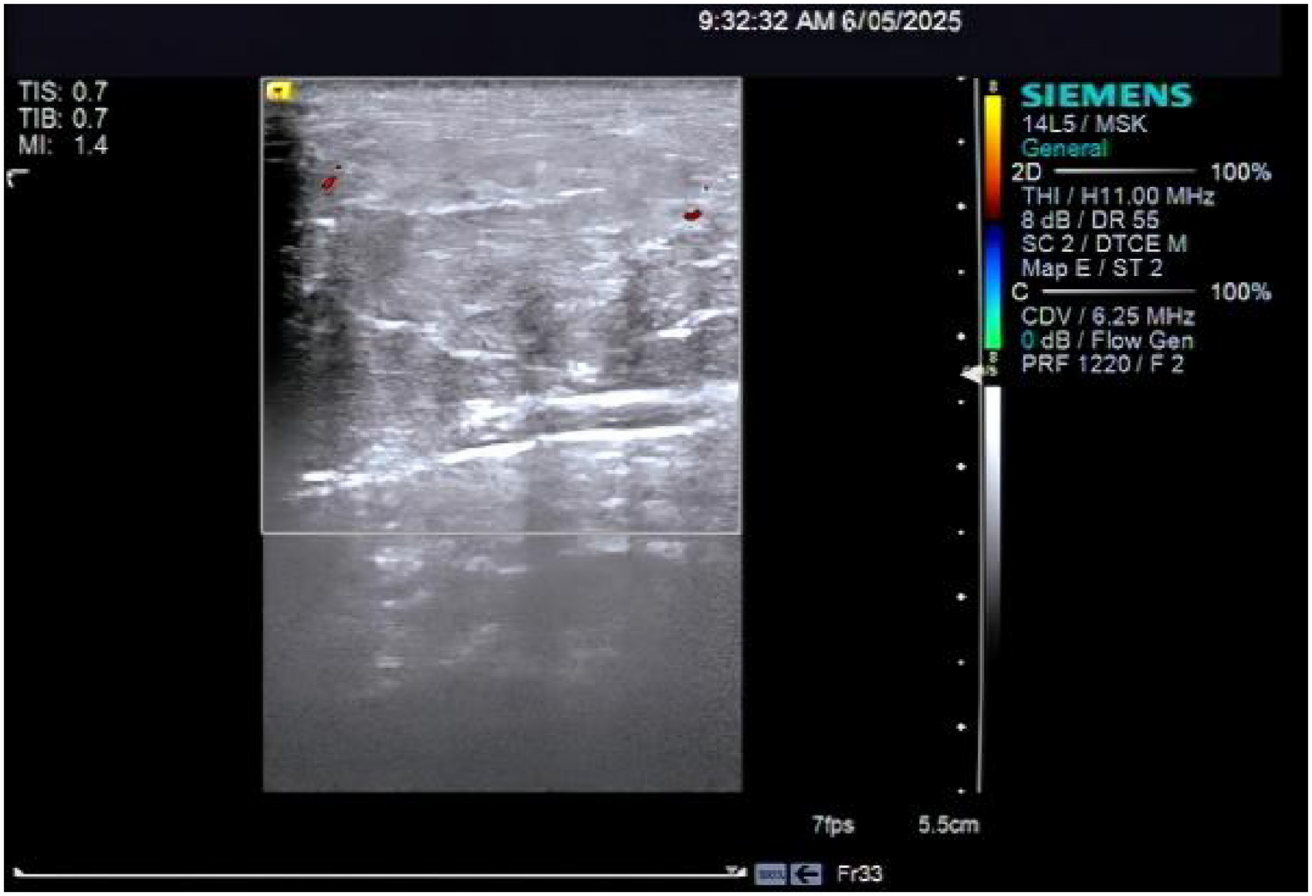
Ultrasound of the superficial tissues of the neck.
